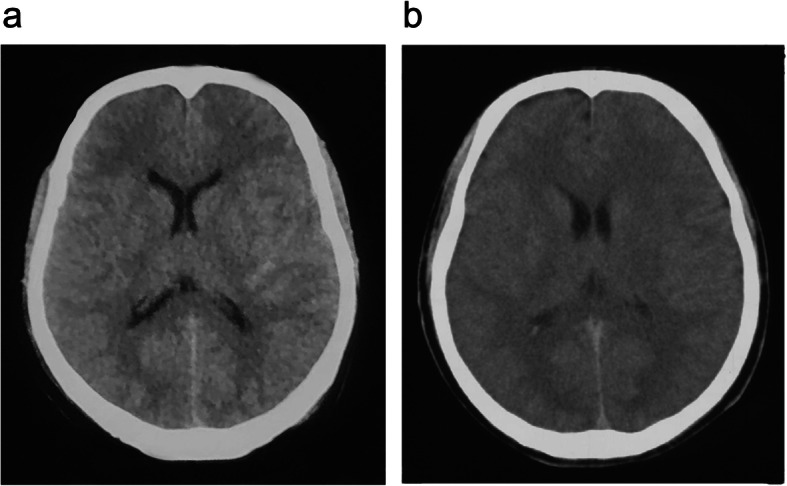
A Head CT obtained 11 h after the accident; the patient was agitated and lost consciousness with convulsions. B The head CT scan obtained 3 days after the accident, which indicated brain swelling
